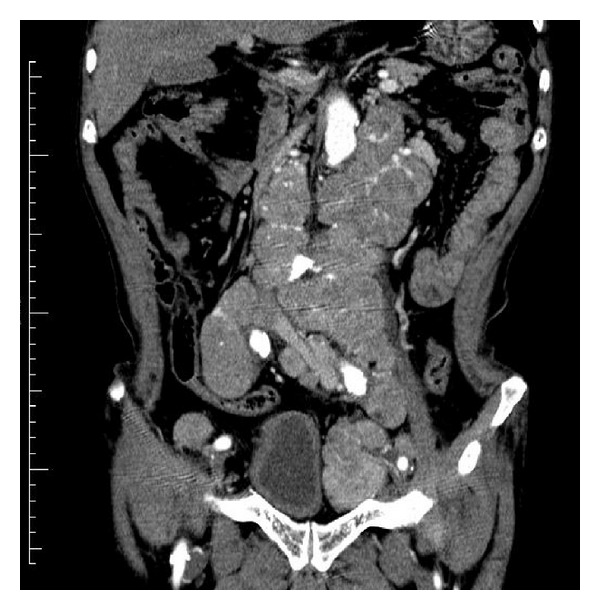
Abdominal computed tomography showing para-aortic and pelvic lymph node enlargement.
